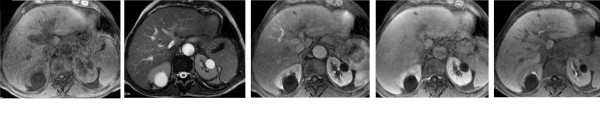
Six months follow up with MRI before and after injection of Primovist: 3a, T1-weighted; 3b, T2-weighted; 3c, arterial phase; 3d, portal venous phase; e, hepatobiliary phase. At six months of treatment, the liver injury is no longer recognizable at MRI examination and the left portal branch is fully recanalized, as with almost complete response to treatment.
